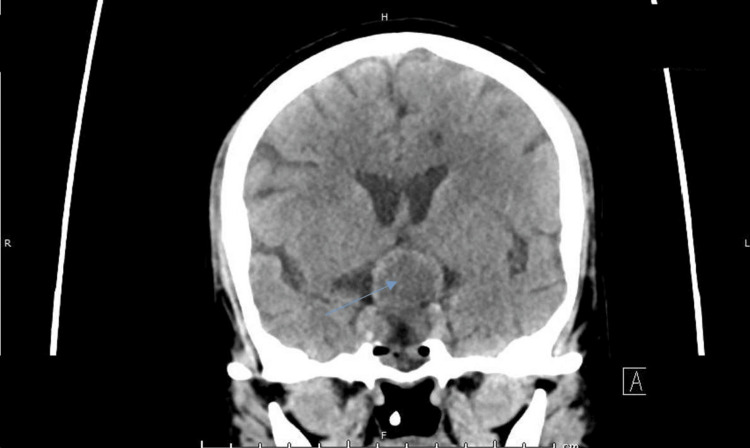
Follow-up non-contrast computed tomography (NCCT) of the brain in the coronal plane obtained 10 days after presentation demonstrating interval cystic degeneration of the previously identified sellar–suprasellar pituitary macroadenoma (arrow).
